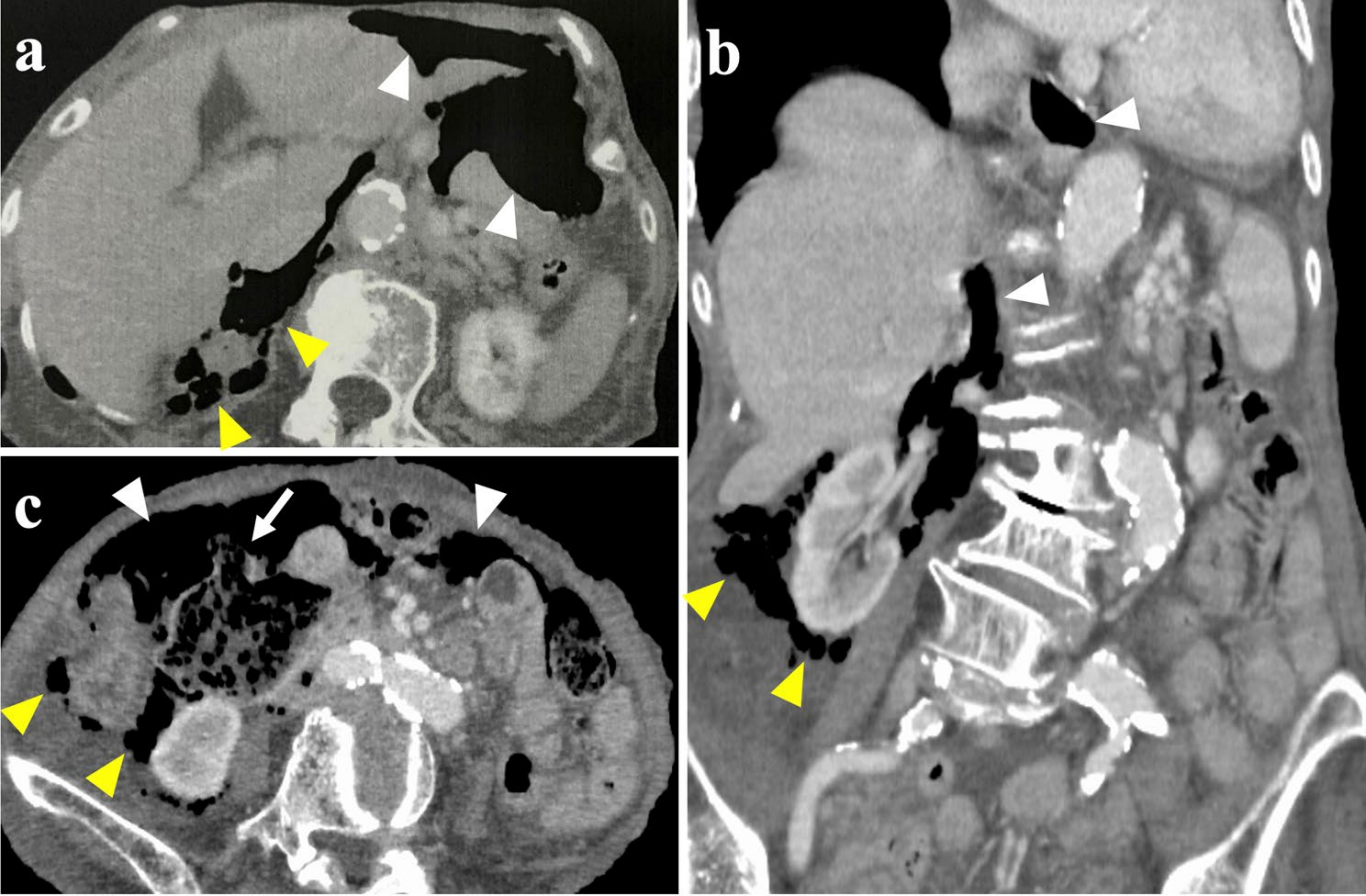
Contrast-enhanced computed tomography (CT) at patient referral to our department. a, b The axial and coronal CT images revealed air in the abdominal cavity (white arrowheads) and right retroperitoneum (yellow arrowheads) and c residue into the abdominal cavity (white arrow) (color figure online)
